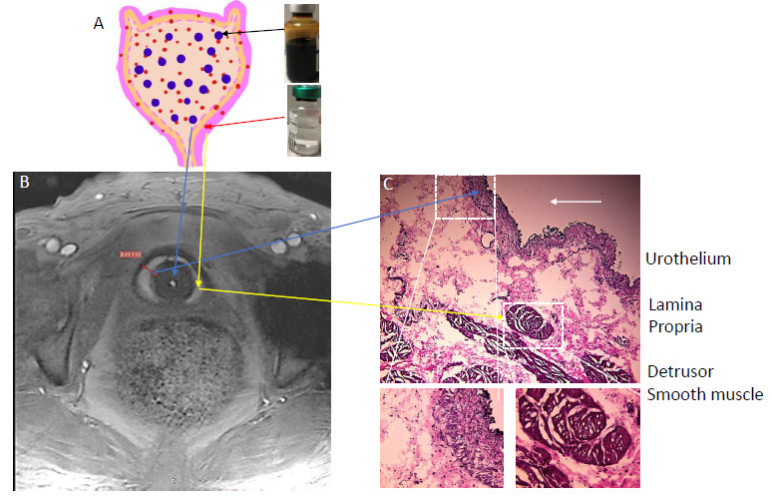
Contrast-enhanced magnetic resonance imaging (MRI) relies on the diffusion of gadolinium into the bladder wall. Novel contrast mixture-enhanced MRI relies on the differences in the contrast mechanisms and molecular weight of two US Food and Drug Administration-approved agents (gadobutrol diluted 1:250 and ferumoxytol diluted 1:104) for increasing the contrast resolution of the bladder wall (Panel
